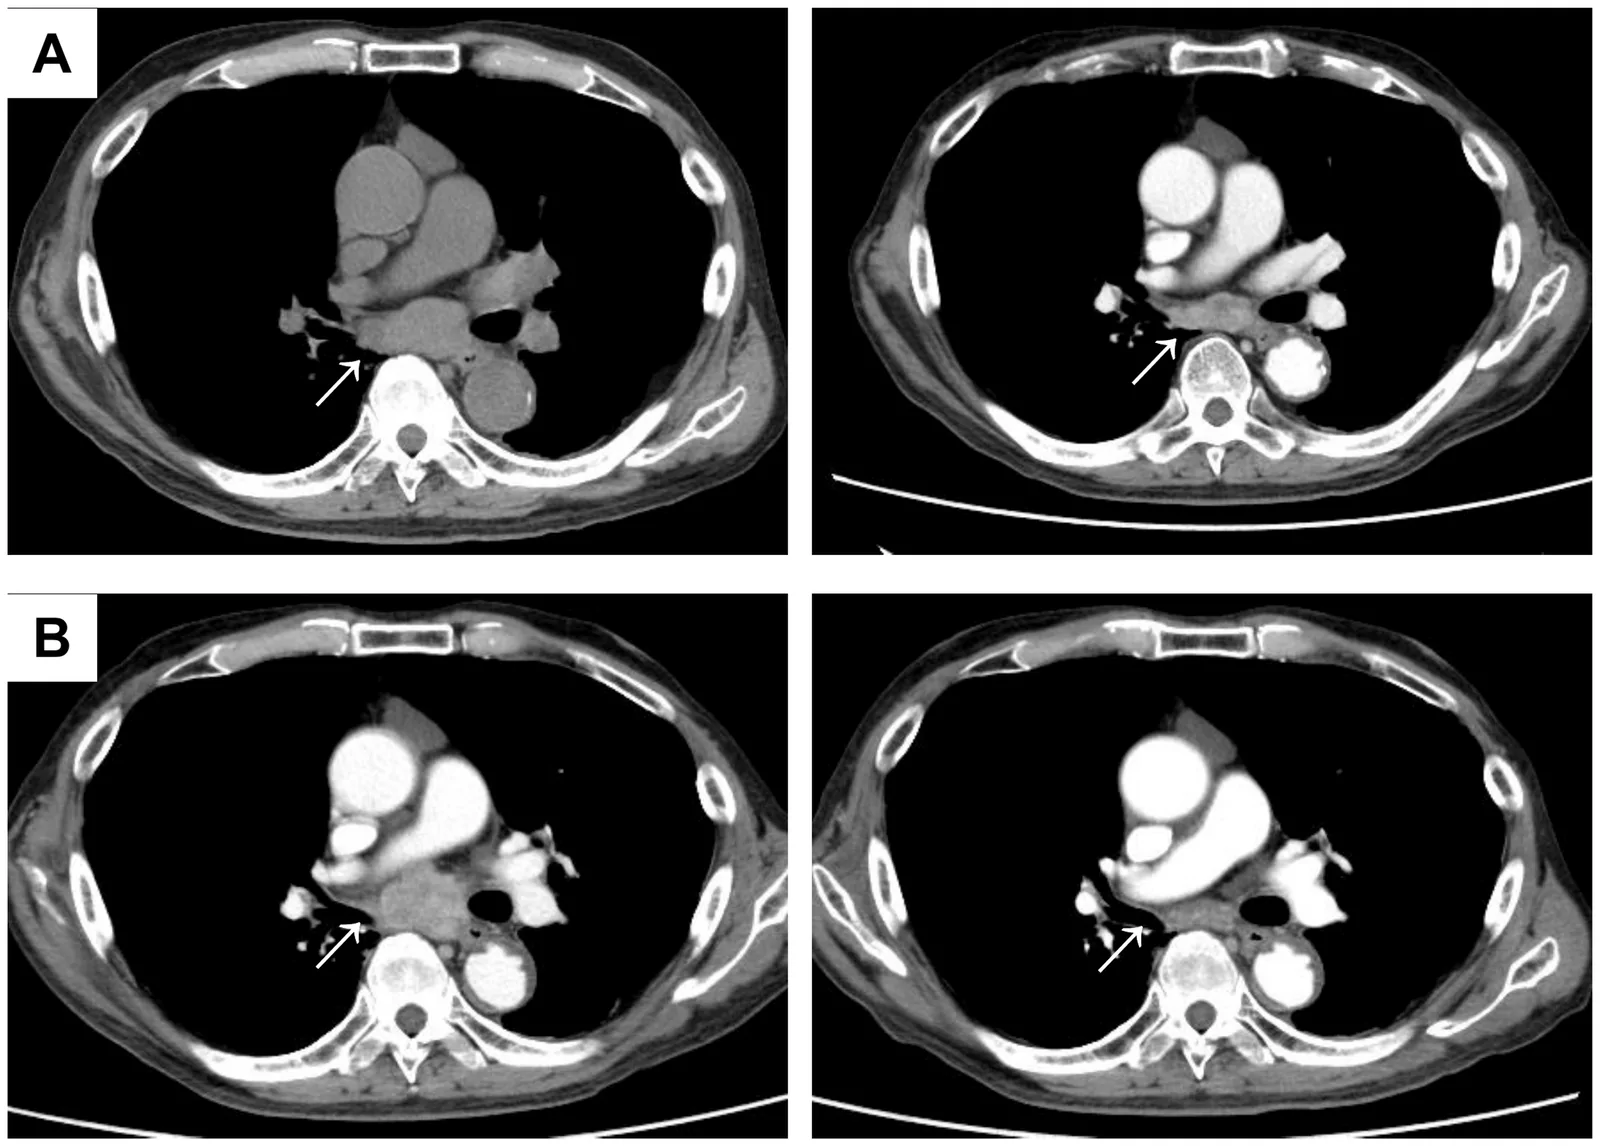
Radiological course of Case 2. (A) Computed tomography images of the subcarinal lymph node lesion before tarlatamab initiation (left) and after the initial tumor response (right). (B) Computed tomography images showing enlargement of the subcarinal lymph node lesion during tarlatamab treatment (left) and tumor reduction after hyperfractionated radiotherapy (right). White arrows indicate the subcarinal lymph node lesion.
